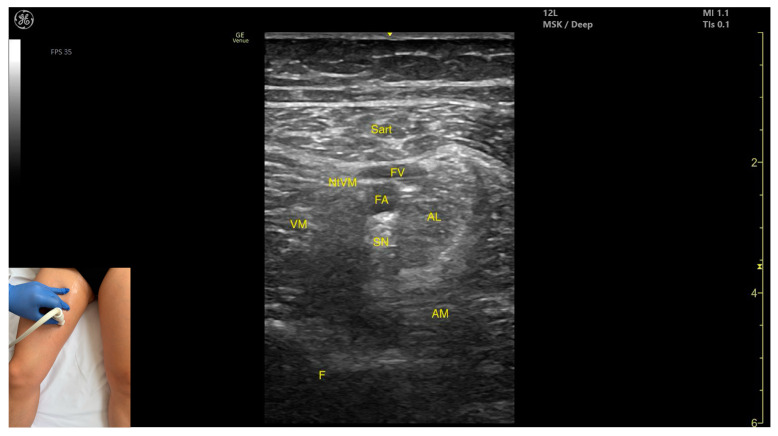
Ultrasound anatomy of *sartorius* (Sart) with key landmarks: Sart—sartorius; FV—femoral vein; FA—femoral artery; SN—saphenous nerve; NtVM—nerve to vastus medialis; AL—*adductor longus*; VM—vastus medialis; AM—*adductor magnus*; F—femur.
